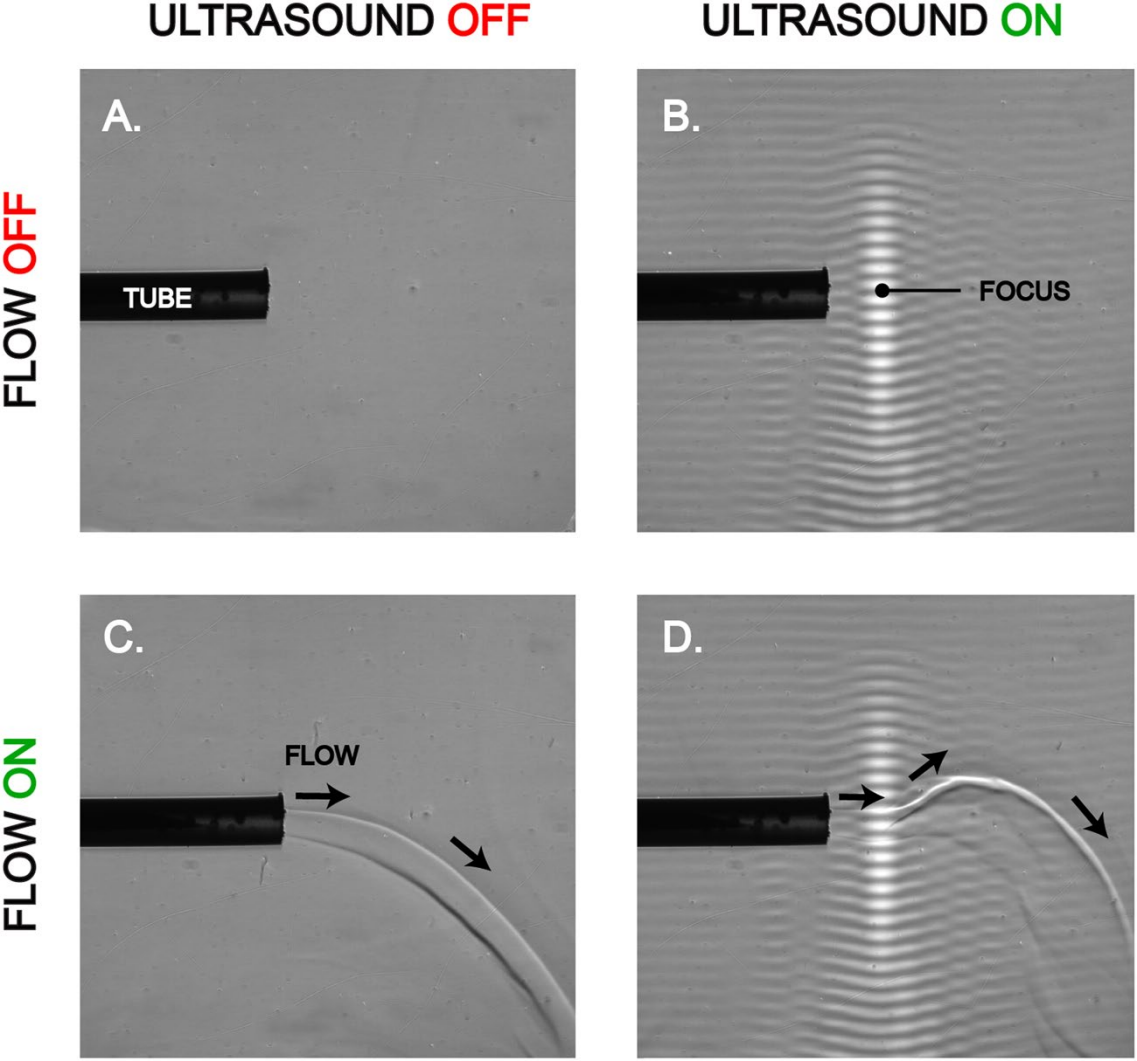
To demonstrate acoustic streaming at the acoustic focus and to simultaneously visualize the acoustic beam, a custom-made Schlieren imaging system was applied. A horizontal flow of cooled water was applied through a nozzle into a water bath to visualize acoustic streaming. The sound is propagating upwards. (A) Ultrasound on, horizontal flow off. (B) Ultrasound on, horizontal flow off. (C) Ultrasound off, horizontal flow on. (D) Ultrasound on, horizontal flow on. When ultrasound is applied simultaneously with horizontal flow, the direction of liquid flow is changing due to upward acoustic streaming.
